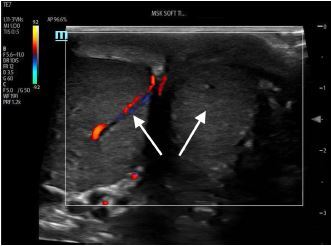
Side-by-side view of initial testicular assessment using point-of-care ultrasound. The testicle on the image-right corresponds to the patient’s painful left testicle which was found to be enlarged with surrounding edema and absent Doppler flow. Arrows highlight presence of flow in unaffected testicle and absence of flow in affected testicle.
